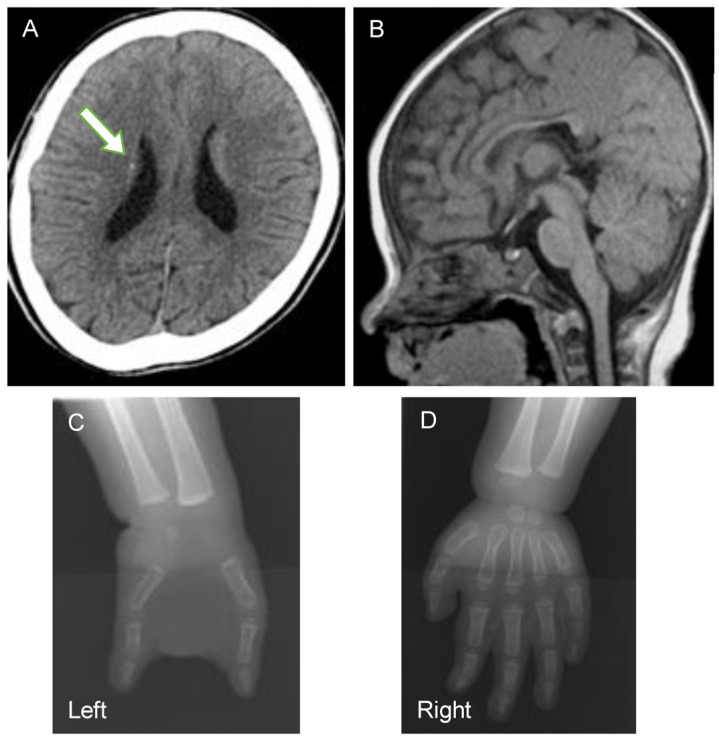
Systemic findings in Case #25 with mutations in the *DOCK6* gene. (A): Computed tomographic (CT) image showing periventricular calcification (*arrow*). (B): Magnetic Resonance Image showing cerebral corpus callosum dysplasia. (C,D): X-ray image of the hands; the left hand (C) has a three-finger defect while the right hand (D) is unaffected.
